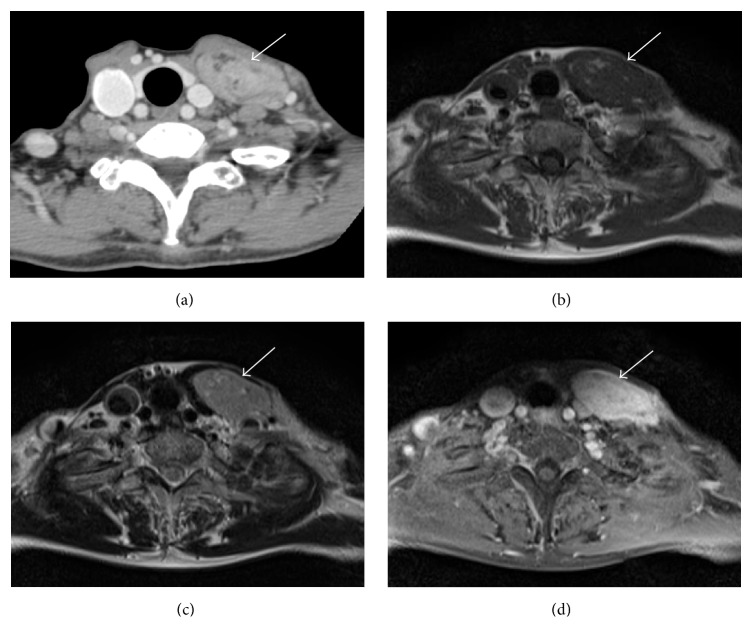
Preoperative imaging findings for Case 1. (a) Contrast-enhanced computed tomography image. T1-weighted axial (b), T2-weighted axial (c), and fat-suppressed gadolinium-enhanced T1-weighted axial (d) magnetic resonance images. Computed tomography and magnetic resonance images show a soft tissue tumor with adipose tissue between the sternocleidomastoid muscle and the anterior strap muscle.
